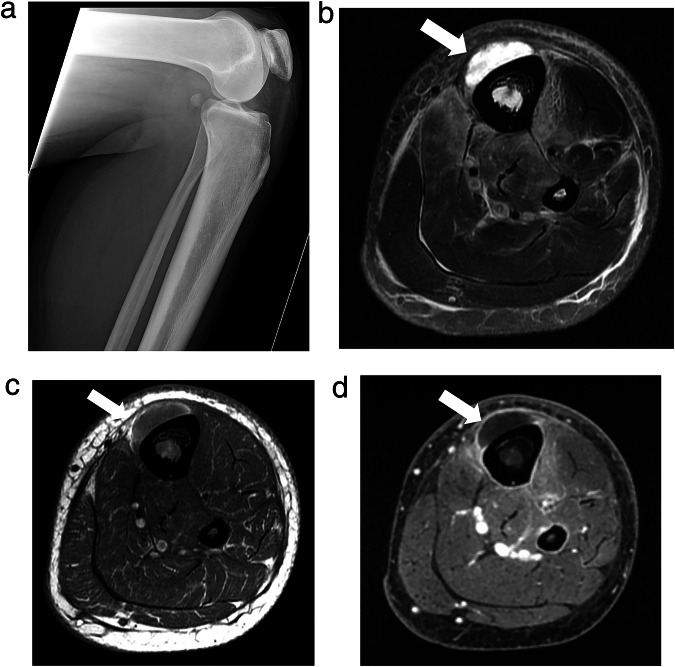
MSKI-RADS Class IV. A 21-year-old woman with left lower leg infection. a Lateral X-ray of the affected left lower leg. b–d Axial fsT2W (water map Dixon), T1W, and axial 3D T1W post-contrast images, respectively showing subperiosteal abscess (arrows) of anterior tibia without confluent T1 marrow signal alteration or enhancement consistent with possible osteomyelitis. The surgical incision, drainage, and biopsy showed fibrous reactive synovial type tissue of the periosteum with patchy chronic inflammation. Bone fragments of the biopsy showed fewer than 2 neutrophils per high-power field
